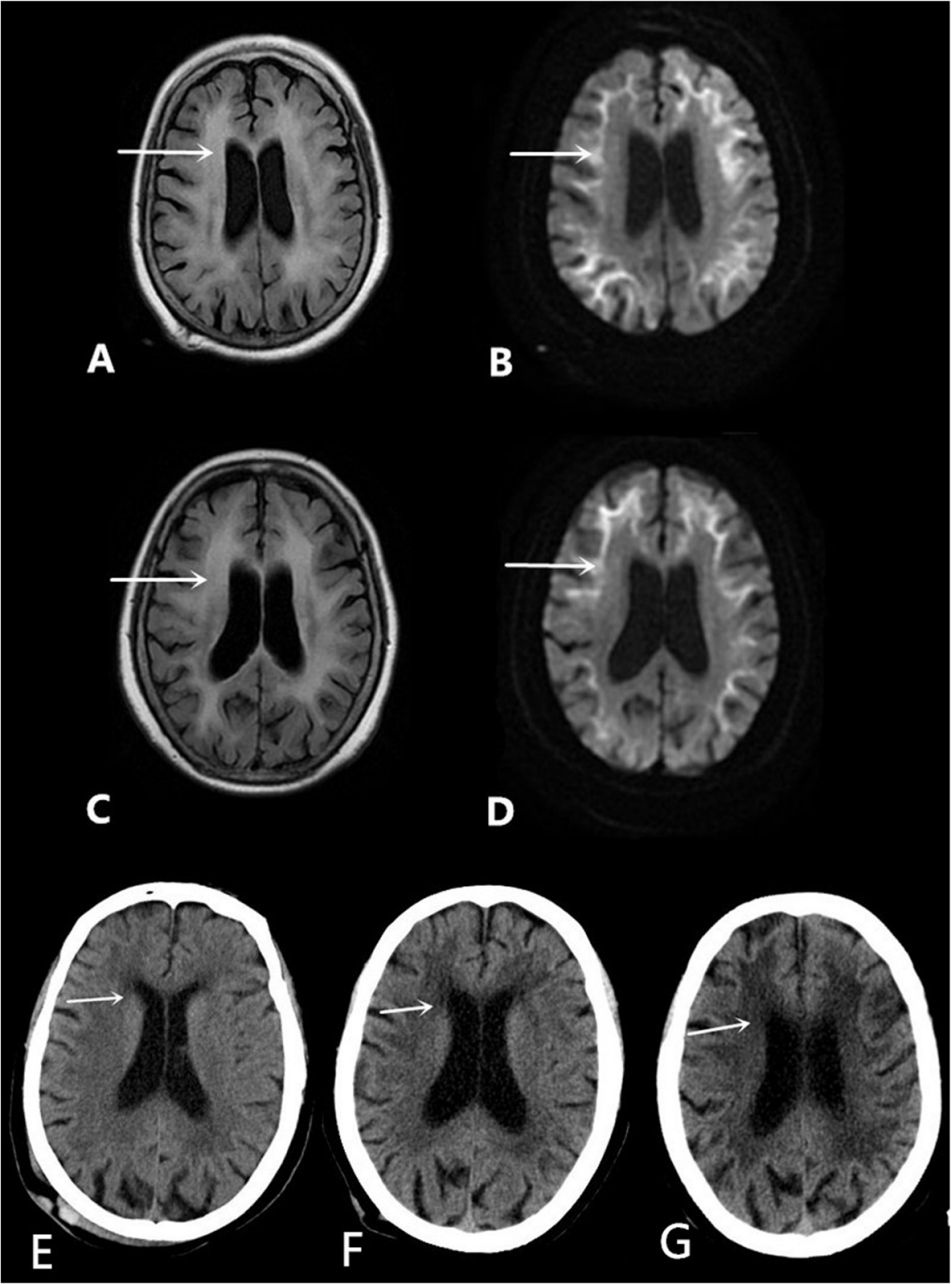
MRI scans on the 5th day after admission: a The T2/FLAIR sequence: the arrow refers to abnormal signals in the white matter region of the brain, b DWI imaging: the arrow refers to high intensity areas in the cerebral white matter and grey matter-white matter junction; the MRI was conducted on the 20th day after admission: c The T2/FLAIR sequence: the arrow refers to abnormal signals in the white matter region of the brain, d DWI imaging: the arrow refers to high intensity areas in the cerebral white matter and grey matter-white matter junction. Cranial computed tomography scans showed a low-density lesion in the white matter, area-brain atrophy, and leucoencephalopathy: e in 2012, f in 2018, and g this hospitalization
